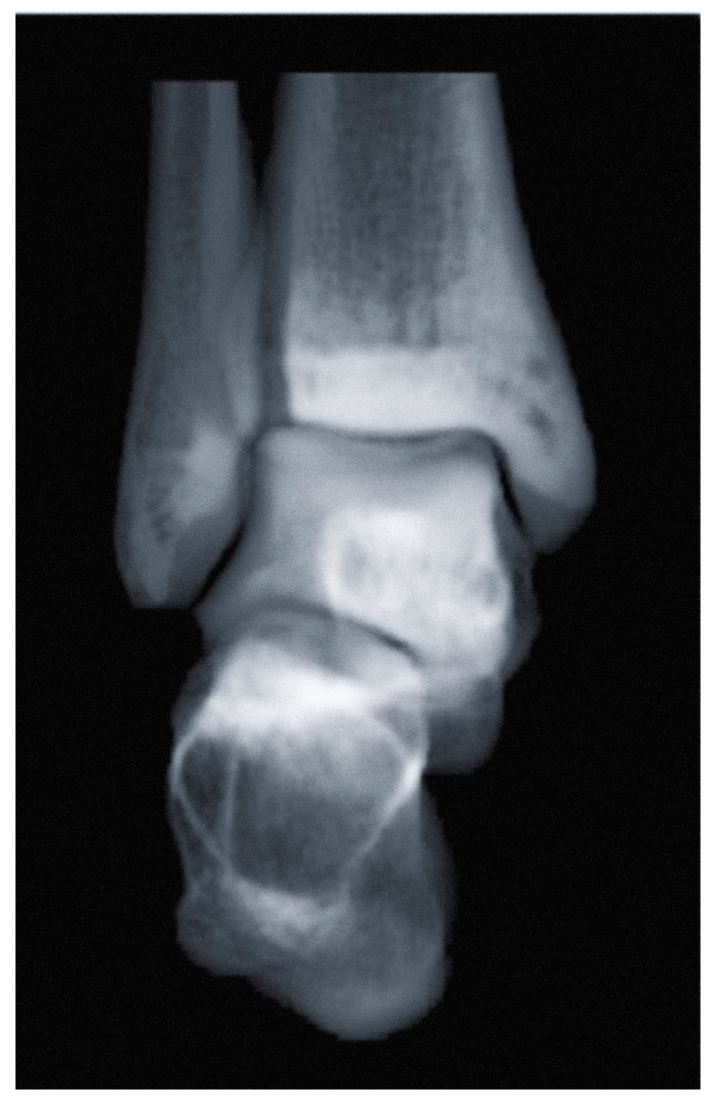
A digitally reconstructed radiograph (DRR) anteroposterior view of the ankle joint.
